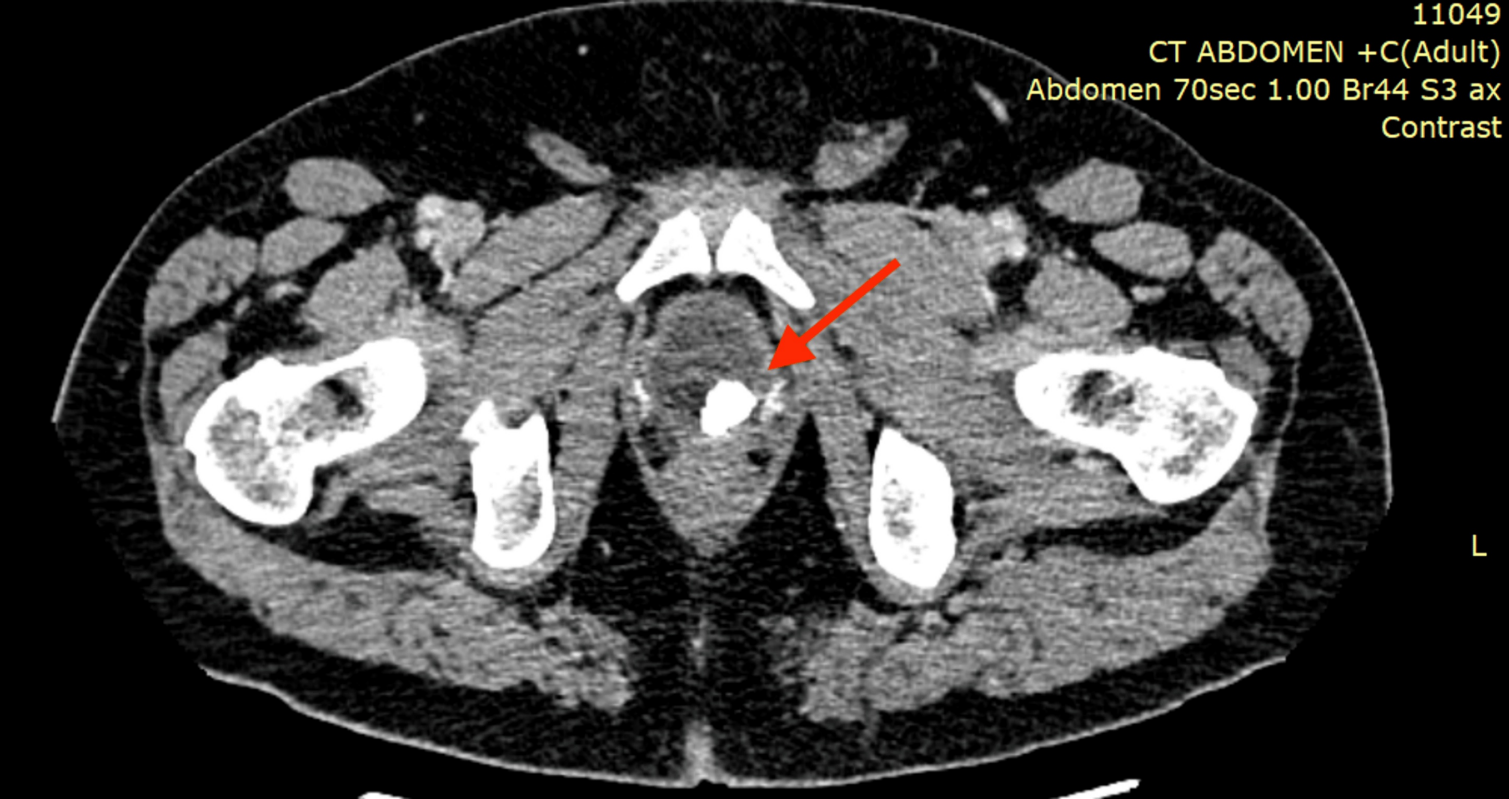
Contrast-enhanced computed tomography (CT) of the pelvis in a transverse plane demonstrating a well-defined hyperdense calcified lesion at the vesicourethral anastomosis (arrow), consistent with a bladder stone formed around migrated Hem-o-Lok® clips.
